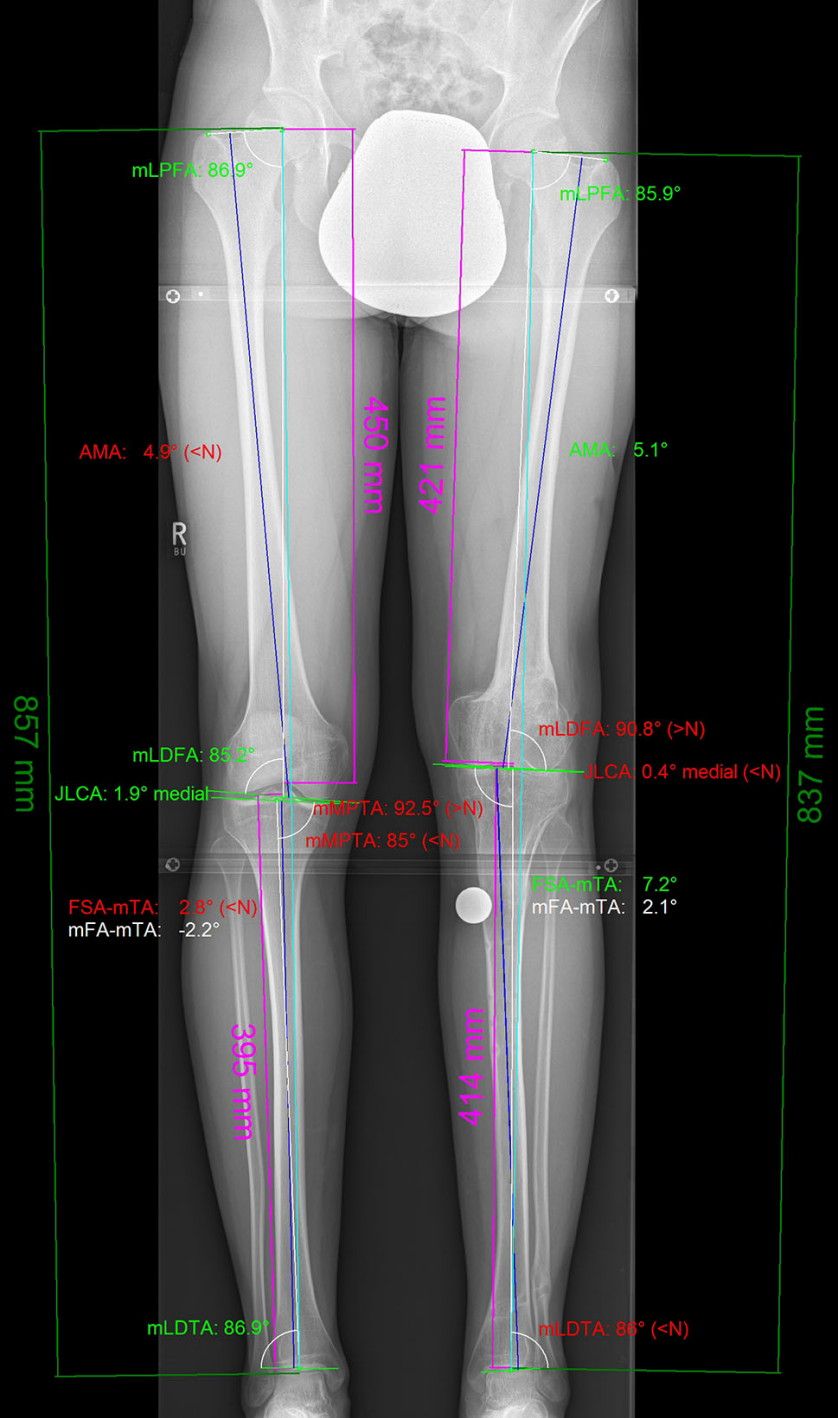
Postoperative full-weight-bearing long-leg radiograph. Analysis of the deformity with the planning software mediCAD (Hectec, Germany), angles according to Dror Paley. Measurements are in Table 2
